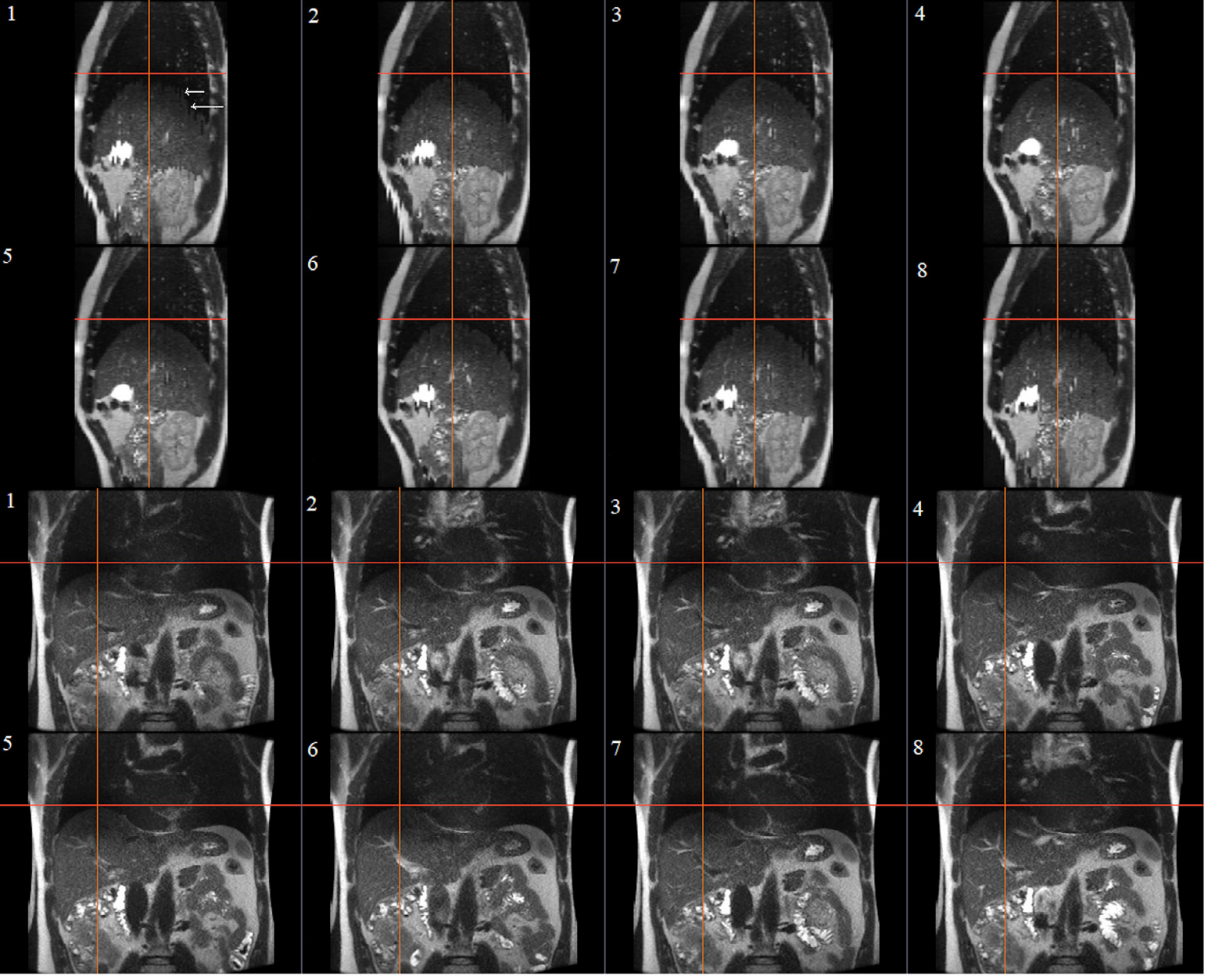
T2‐w SSFSE sagittal (above) and coronal (below) plane MR images from abdomen area (coronal image acquisition) in eight respiratory phases. Images from expiration phases (phase 4,5) has fewer stitching artifacts (marked with arrows at inspiration, phase 1) caused by irregular breathing of volunteer (VOL1). Maximum displacement of the liver dome between the inspiration and expiration phases was 10.6 mm
